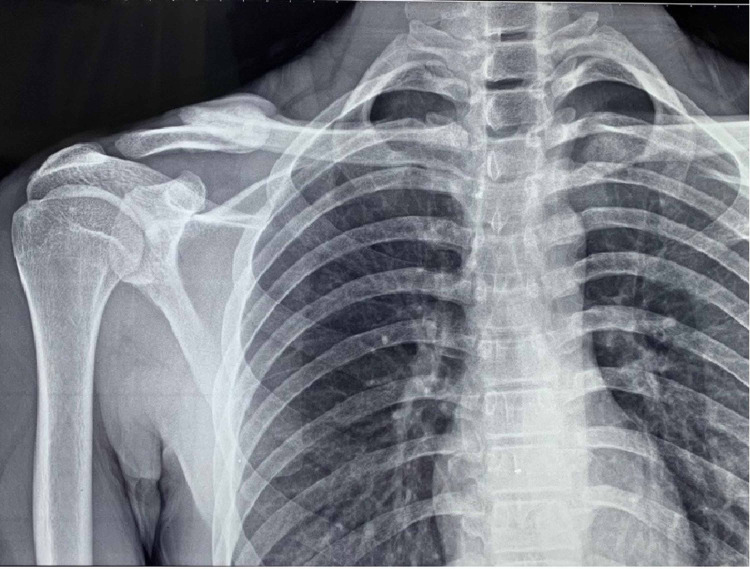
Radiograph showing a right clavicle mal-union with shortening at 12 months follow-up
